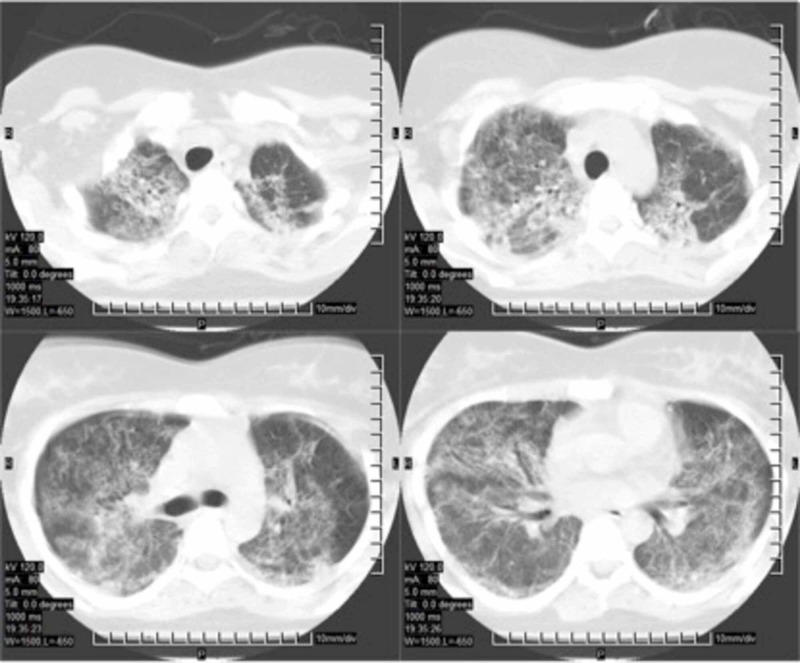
Thorax CT images of the patient before corticosteroid treatment showing bilateral extensive ground-glass opacities with adjacent traction bronchiectasis and interlobular septal thickenings.
